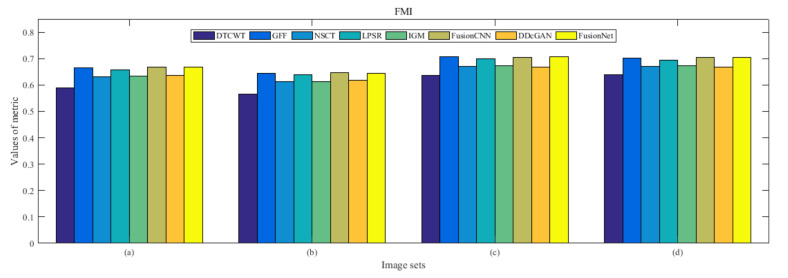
Values of feature mutual information (FMI) in the fused images (MRI-CBF): (a) is composed of eight images in Figure 42, (b) is composed of eight images in Figure 43, (c) is composed of eight images in Figure 44, (d) is composed of eight images in Figure 45.
